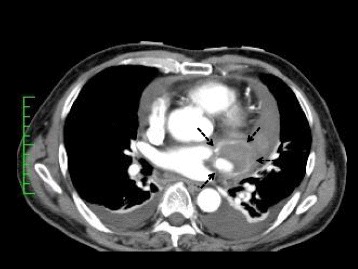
Computed tomography showing pericardial effusion (*solid arrow*) and a coronary artery aneurysm (*dotted arrow*) with an enhanced wall
